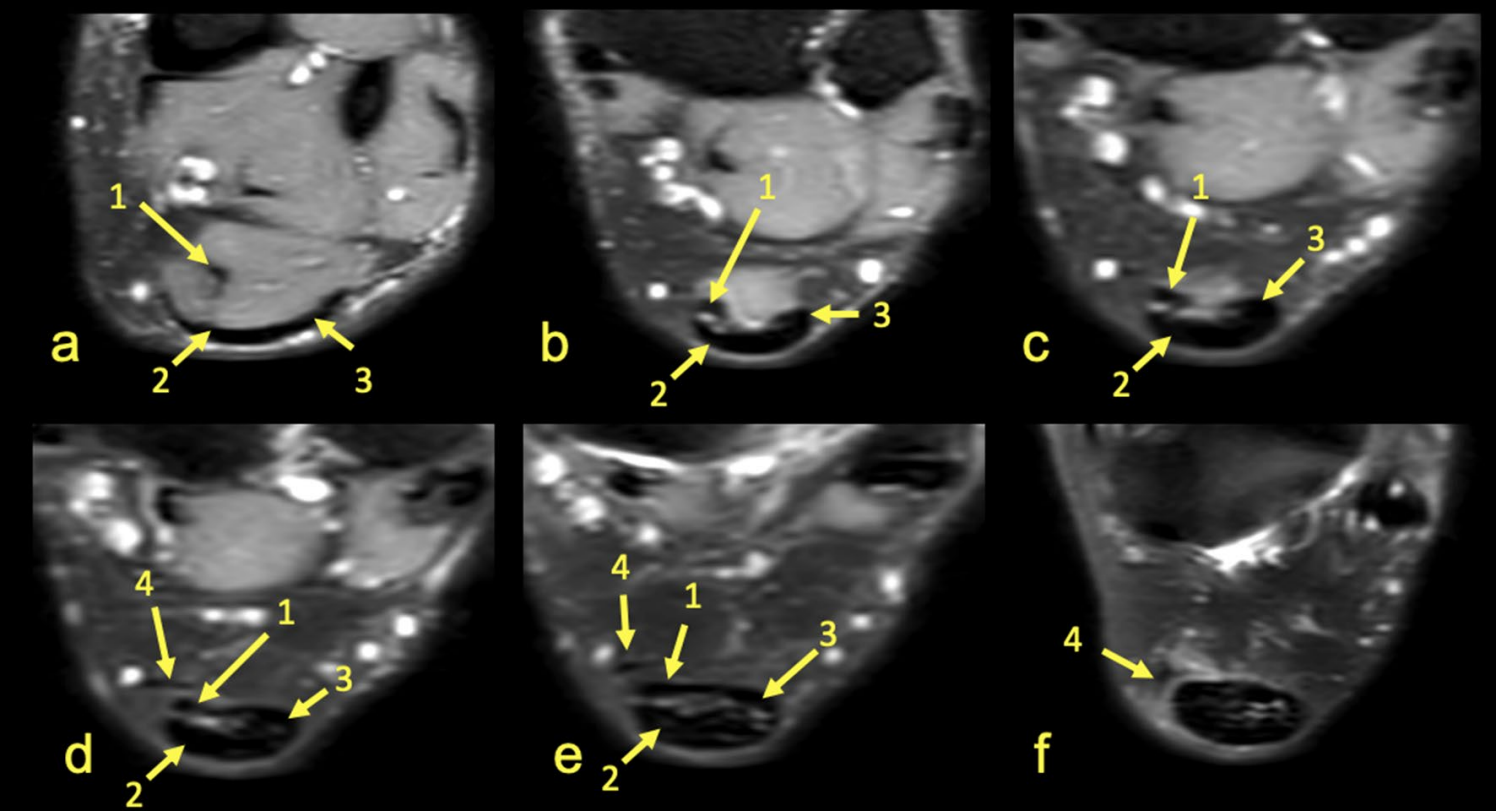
MRI left ankle, PD FS at the level of the distal tibia (a–e), the talus (f); (a)—the highest section, (f)—the lowest section. 1—T-Sol, 2—S-MGC, 3—S-LGC, 4—the plantaris tendon (type 3).
